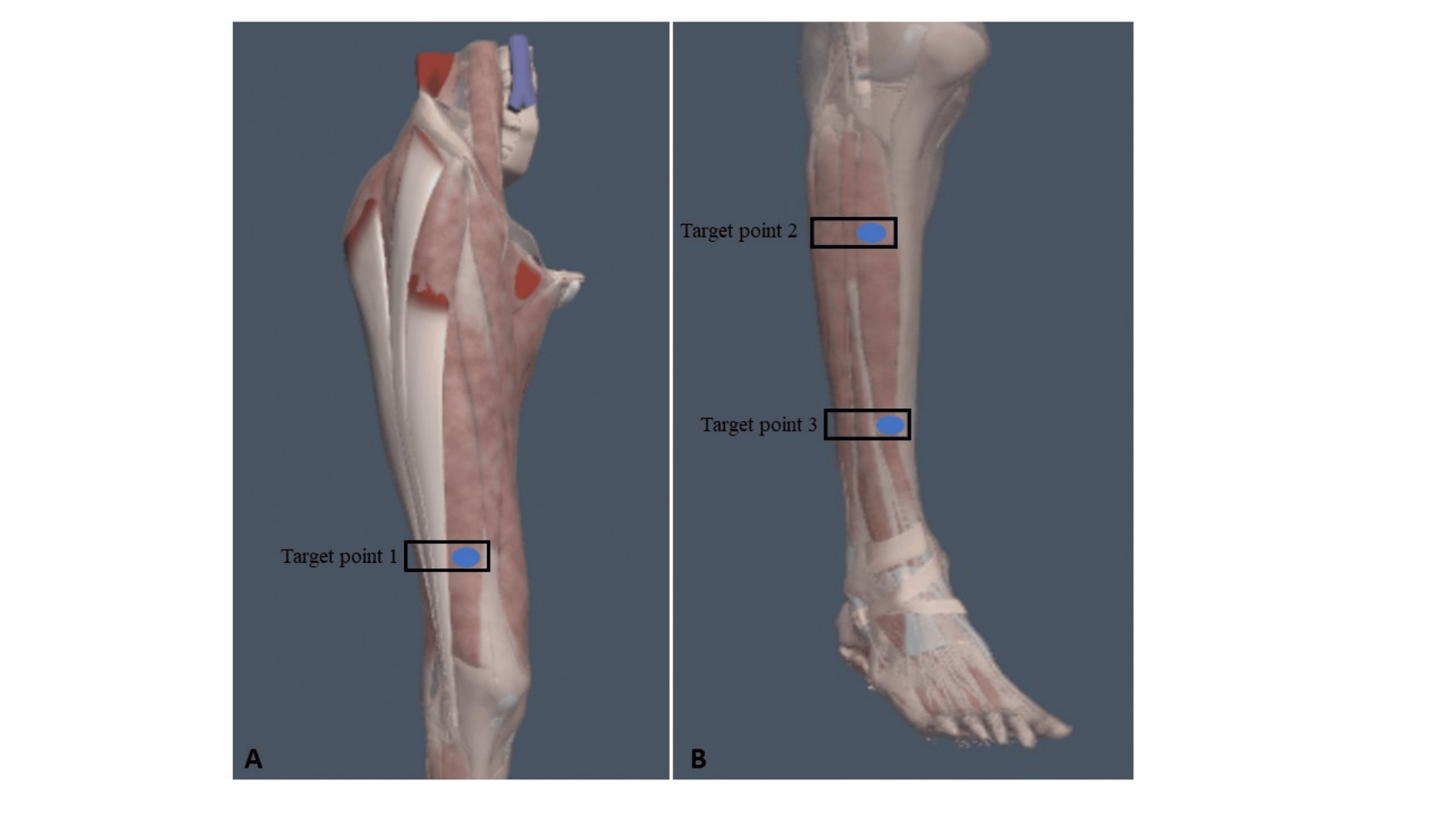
Target points of the ultrasound-guided injection
